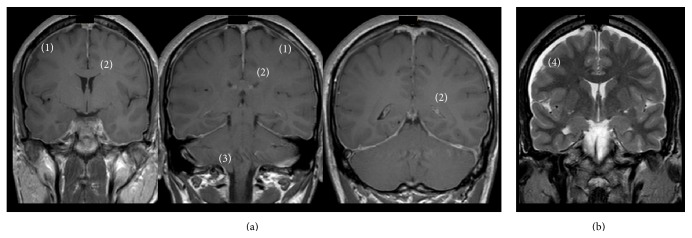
(a) T1-weighted brain MRI scan with gadolinium showing diffuse pachymeningeal enhancement (1), decrease in ventricle size (2), and cerebellar tonsil descent (3). (b) T2-weighted brain MRI scan showing subdural fluid collections (4).
